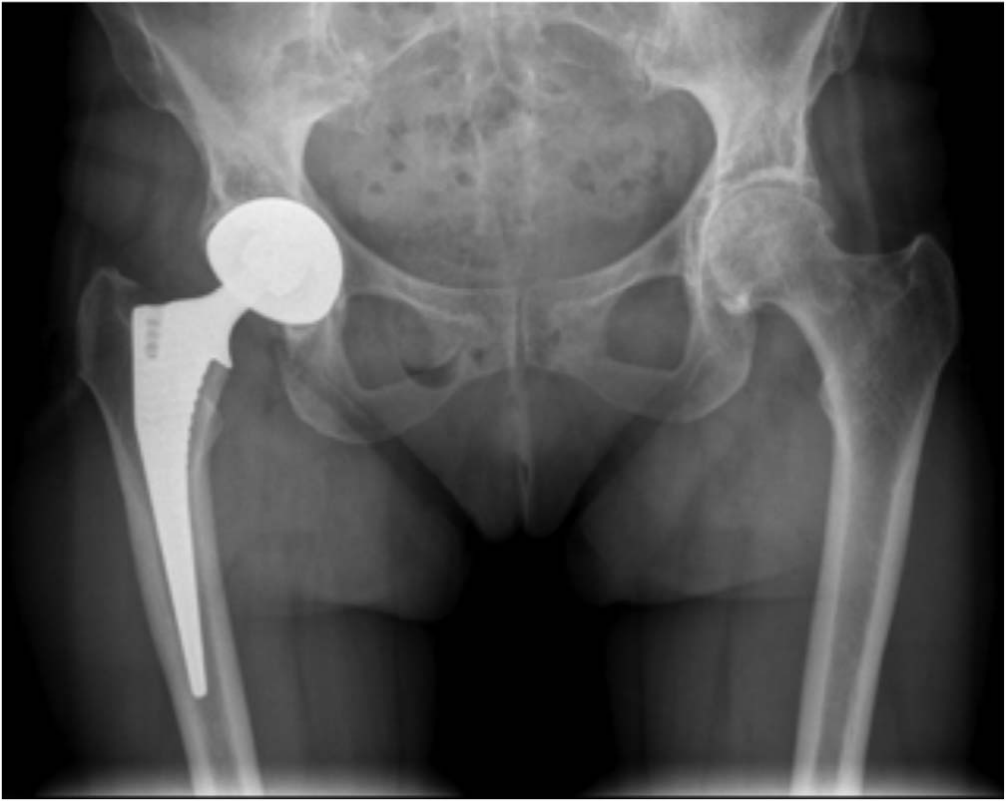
Anteroposterior radiograph of the pelvis showing the right Total Hip Arthroplasty.
